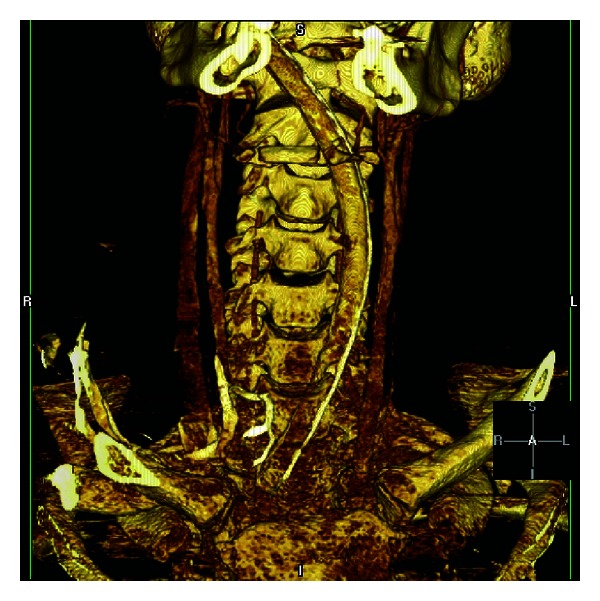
Three-dimensional CT scan of neck.
